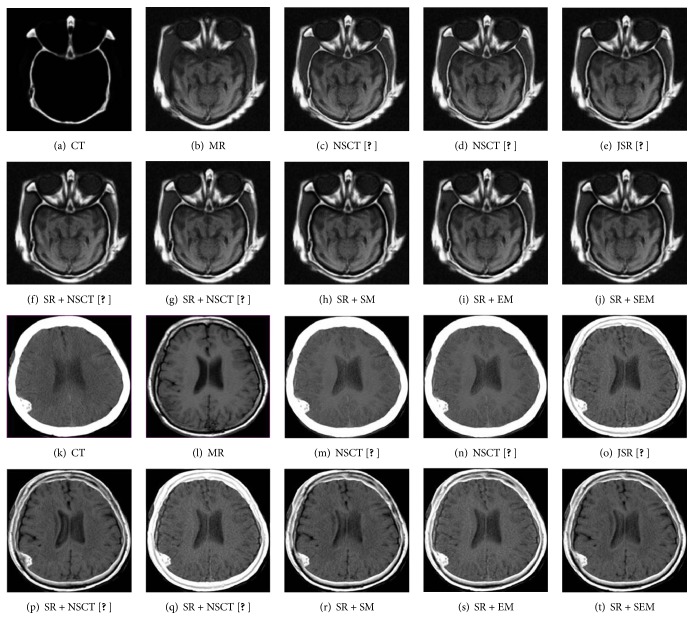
The CT and MR image fused results of different fusion methods.
